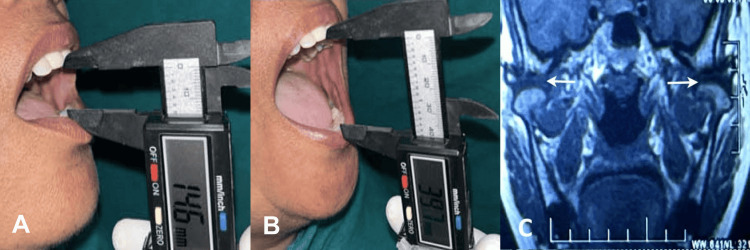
(A) Preoperative mouth opening (14.6 mm), (B) postoperative mouth opening (39.7 mm) at the three-month follow-up, and (C) MRI scan showing partial adhesion of the bilateral temporomandibular joint.
